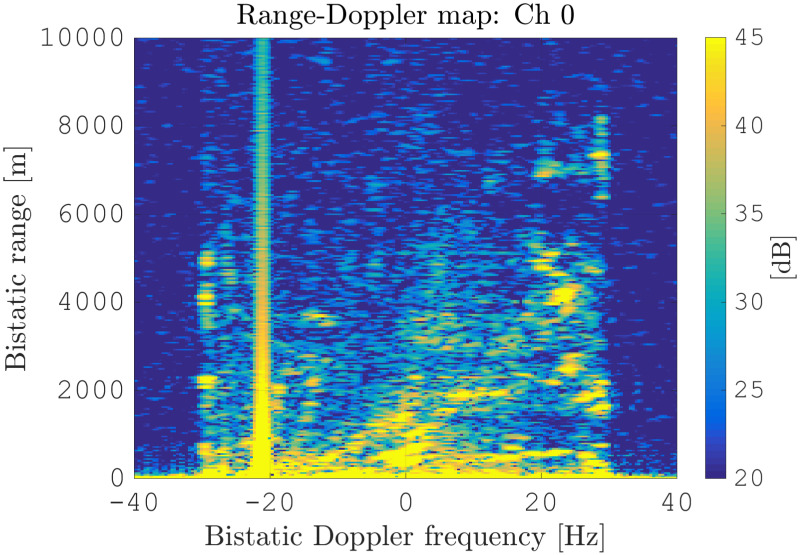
Initial range-Doppler map for the leading antenna.
